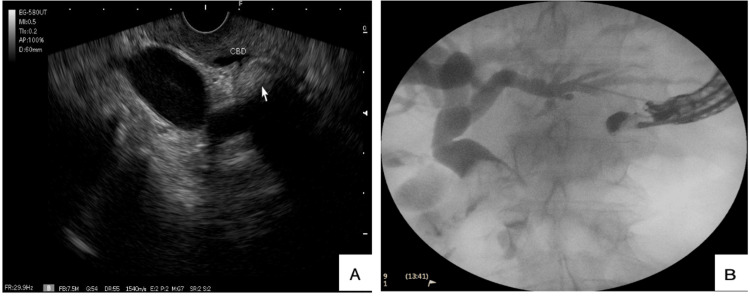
Patient with type IV MS with total cholecystobiliary fistula and multiple stones identified by fluoroscopy, causing dilation of intrahepatic and extrahepatic biliary ducts. The patient required BER-HJ. A) Visualized stones on EUS with dilation of the biliary tract. The white arrow points to stones within the bile duct. B) Antegrade cholangiography via EUS showing multiple stones in the CBD. Abbreviations: MS, Mirizzi Syndrome; CBD, Common Biliary Duct; EUS, Endoscopic Ultrasound; BER-HJ, Bilioenteric Reconstruction with Hepaticojejunostomy.
